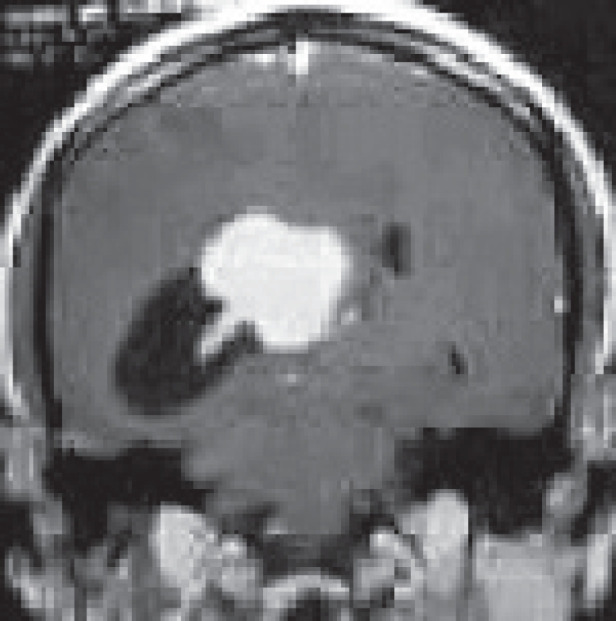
Pre-op MRI Brain, T2W coronal section showing a well-defined lobulated hyperintense mass lesion in atrium of the right lateral ventricle causing mass effect in form of dilated occipital horn of right lateral ventricle.
